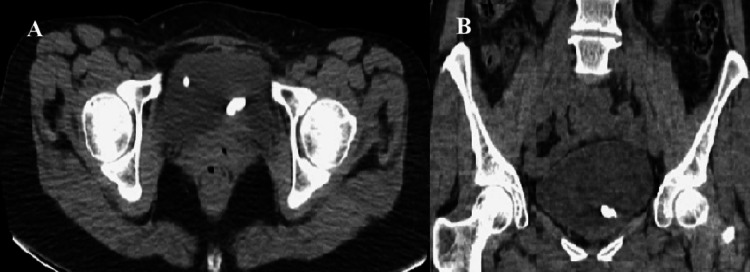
Computed tomography image demonstrating bladder stones. (A) Axial section and (B) coronal section.
